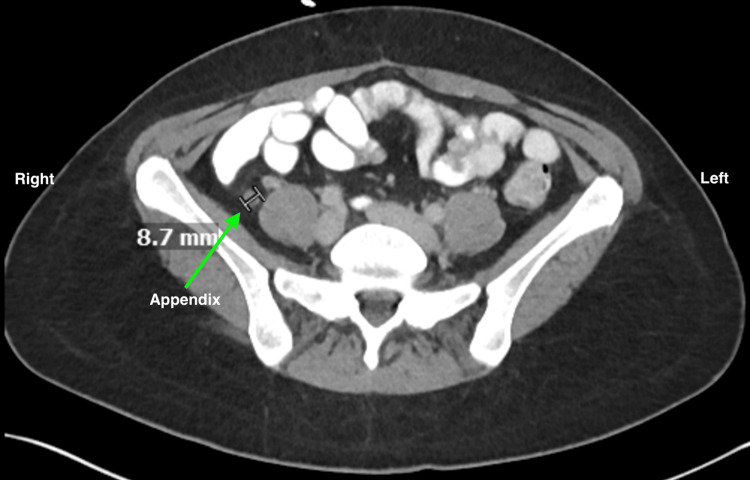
Axial CT scan demonstrating a dilated appendix, with inflammation surrounding the tip, measuring 8.7 mm (arrow). CT, computed tomography
